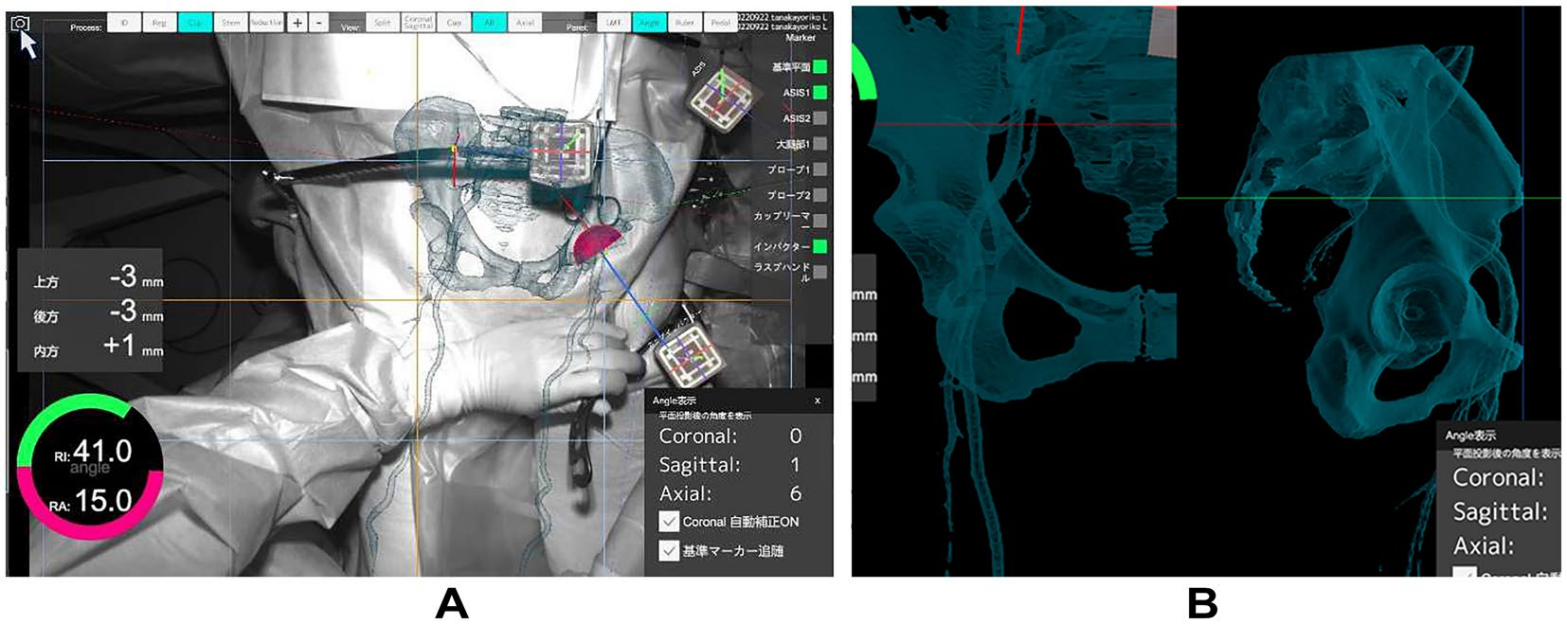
Screens of the augmented reality (AR) computed tomography (CT)-based navigation system are shown. (A) Surgeons can view a three-dimensional model of the pelvis on the real surgical field on the monitor. Radiographic inclination (RI) and radiographic anteversion (RA) of the cup are provided in real time. (B) Vessel locations can be displayed during surgery.
